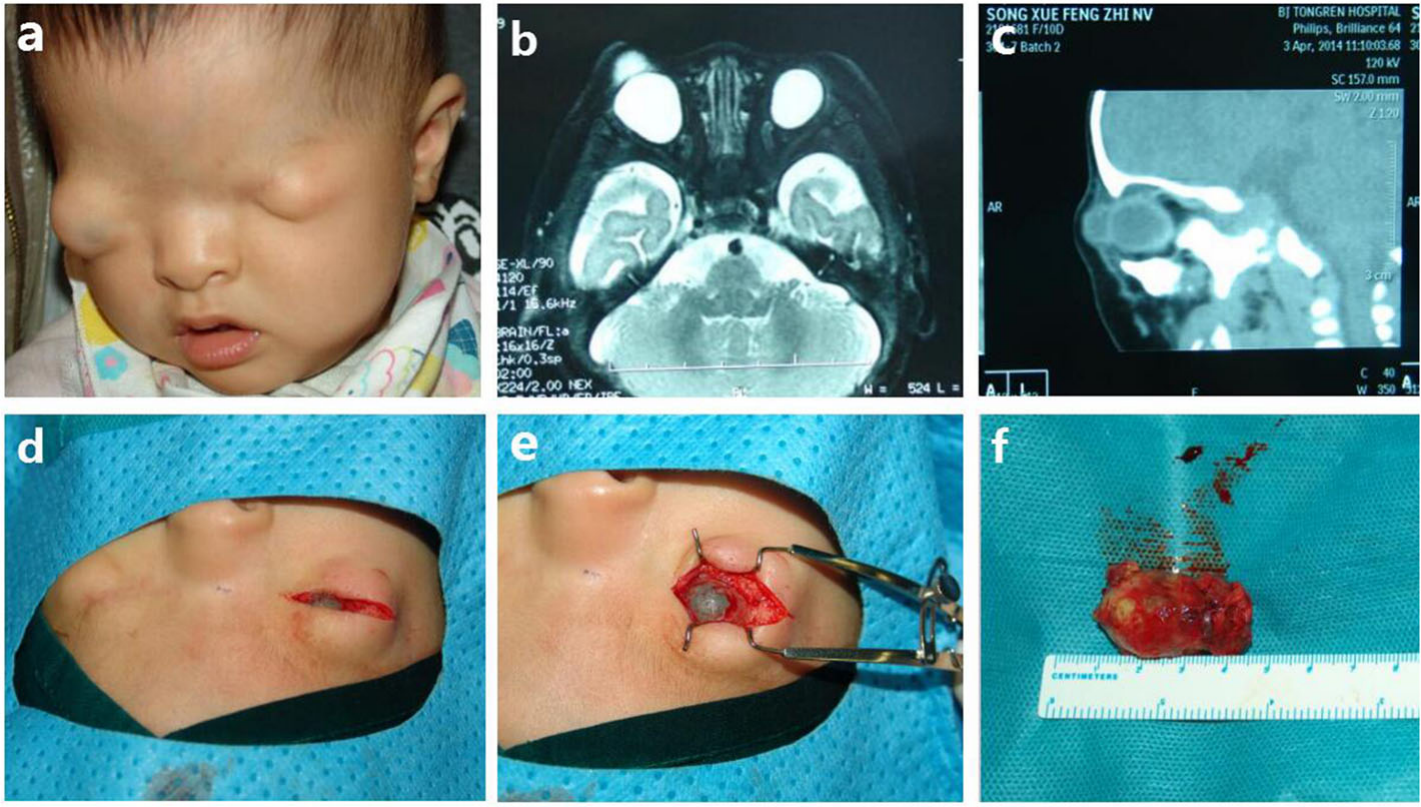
a An eleven-month-old girl with complete cryptophthalmos bilaterally and proptosis in the right side. b MRI shows an enlarged, bulging globe with anterior cyst in the right side. c CT scan displays right eye proptosis with anterior cyst. d-e Intraoperative photograph shows a large cystic globe. f Enucleation of enlarged cystic eyeball
